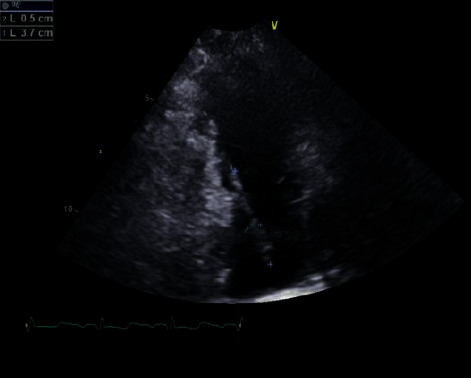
Transthoracic echocardiogram revealed extensive mobile 3.7 × 0.5 cm vegetation in the pulmonary valve with moderate to severe pulmonic regurgitation mobile 3.7 × 0.5 cm vegetation in the pulmonary valve with moderate.
